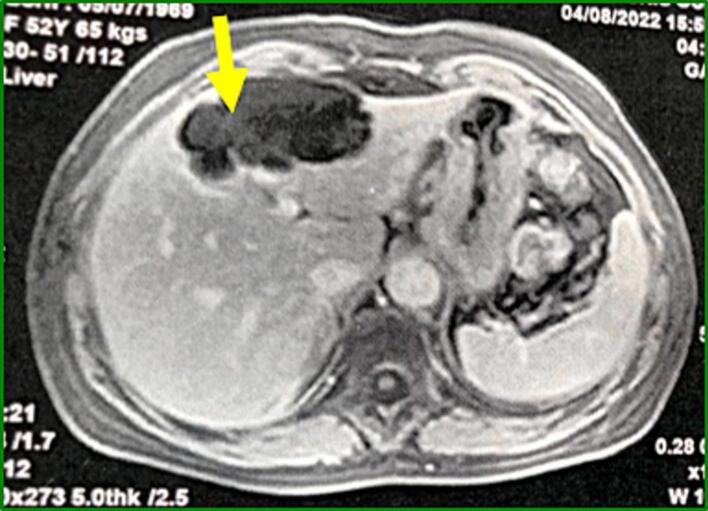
T1-weighted sequences of hepatic MRI revealing a hypointense, fluid-dense mass without clean wall and dotted by fine septa (yellow arrow).
